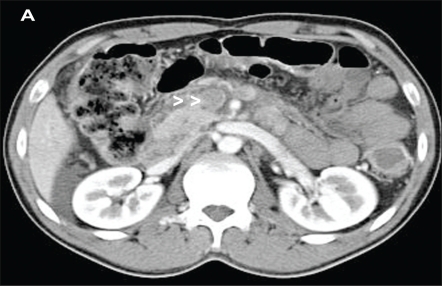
Contrast-enhanced axial computed tomography scan shows clot in superior mesenteric vein (A, arrow heads), portal vein (B, right arrow heads) and splenic veins (C, left arrow heads) in a patient with polycythemia rubra vera and mobile right heart thrombus.
